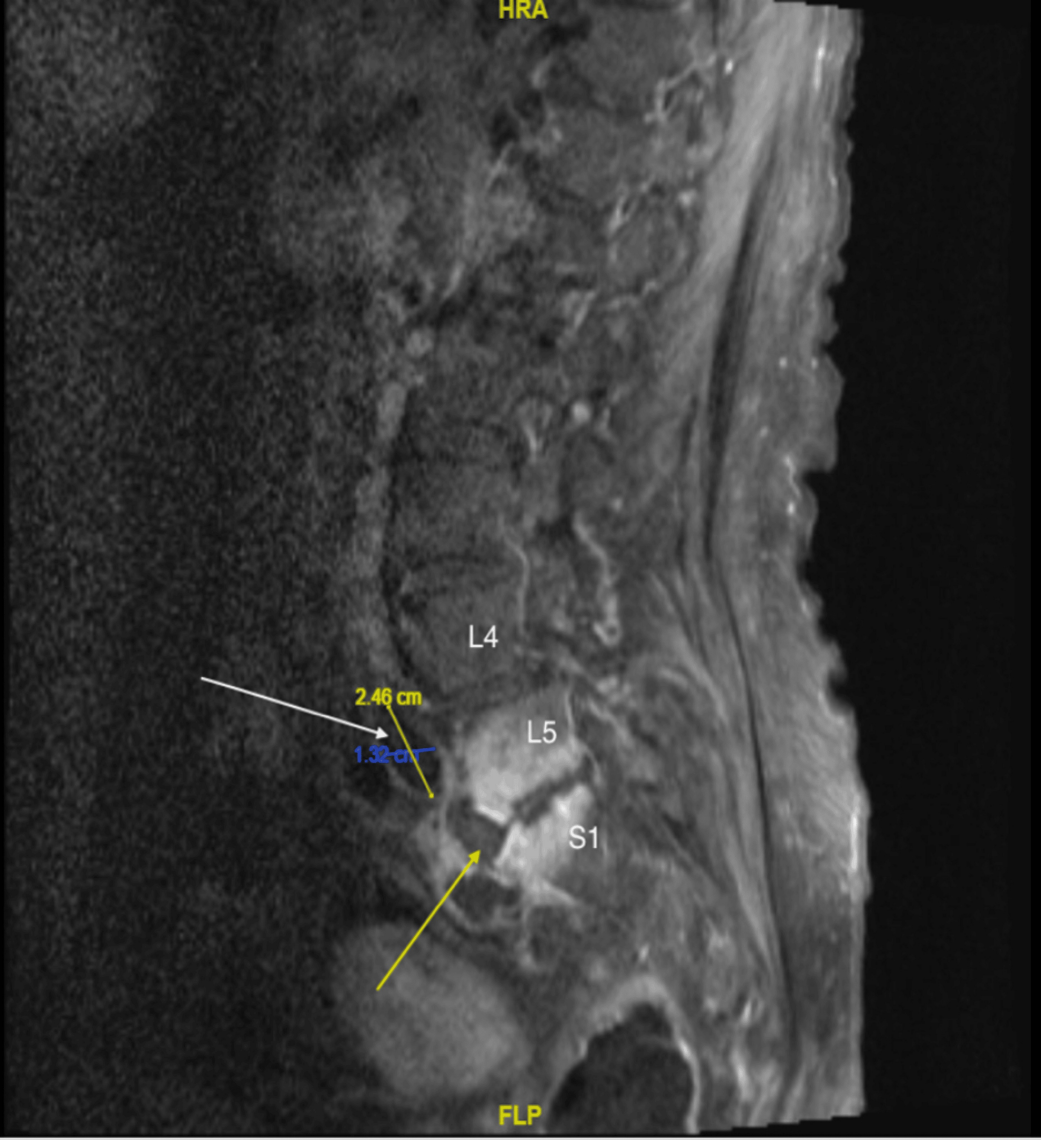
MRI of the lumbar spine showed a small abscess within the soft tissue (white arrow) along the ventral margin of the intervertebral disc and the anterior cortex of the sacrum, left of midline. Contrast enhancement of the endplates at L5-S1 is consistent with discitis (yellow arrow). The study was limited due to motion artifact MRI: magnetic resonance imaging
